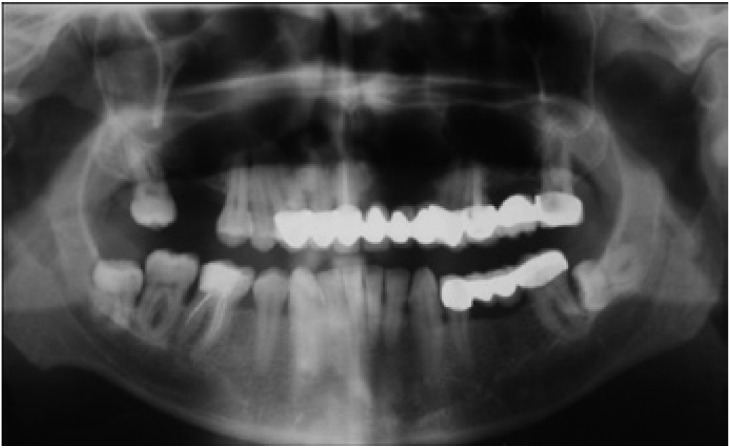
Panoramic radiograph of a KCOT occupying the right maxillary sinus. Note that the border is not readily apparent.
